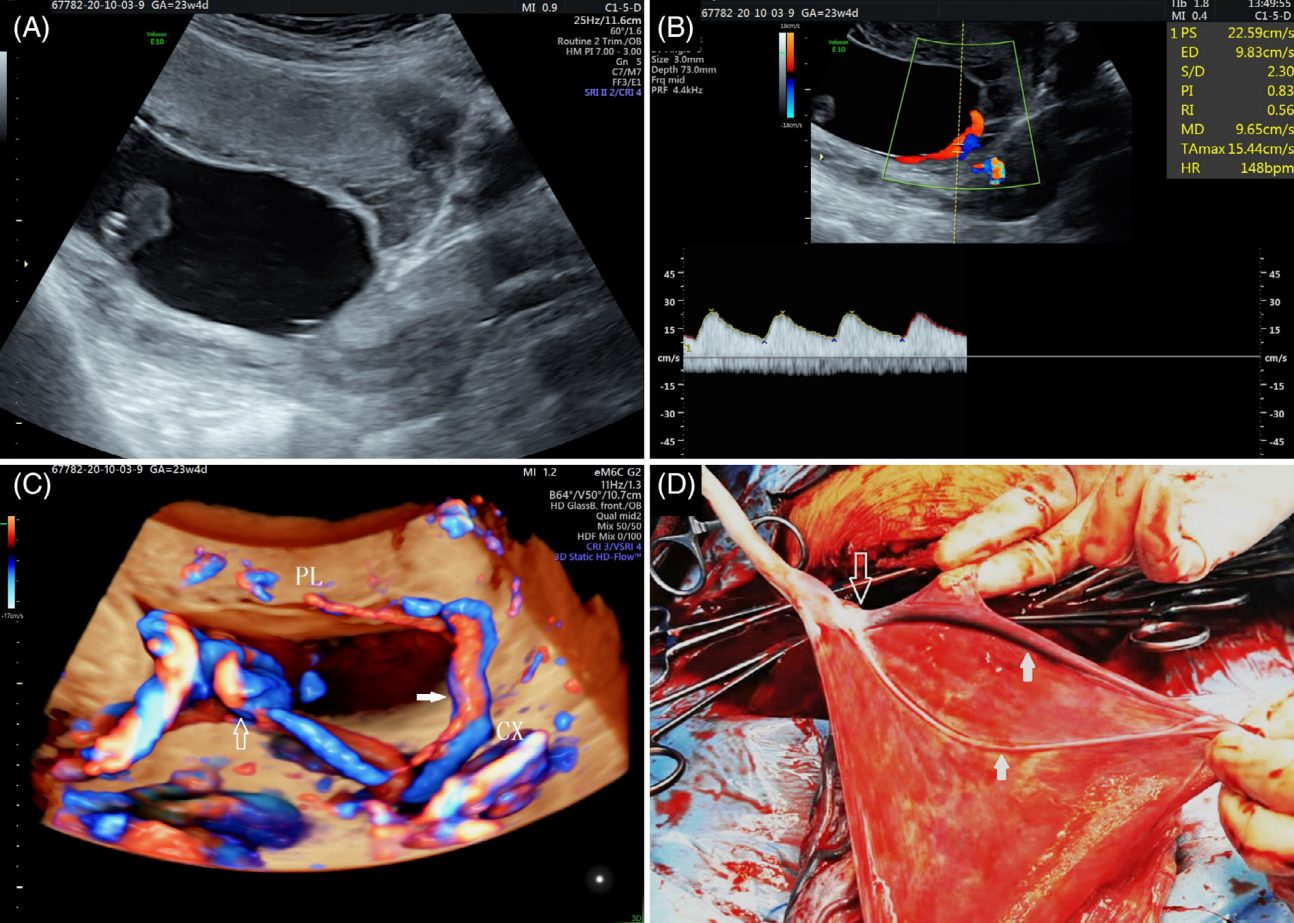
Two‐dimensional ultrasound examination showing short tubular hypoechoic structure near the cervical os suggestive of vessel previa (A). Color Doppler imaging demonstrating arterial and venous blood flow spectrum at the cervical os (B). Flow HD glass body of the umbilical cord showing sail‐like insertion and vessel previa crossing the cervical os and entering the placenta (C). PL, placenta; CX, cervix; hollow arrow, umbilical cord insertion point; solid arrow, sail‐like vessel. Postpartum image of placenta, umbilical cord, and umbilical vessels showing sail‐like insertion point (D). PL, placenta; hollow arrow, umbilical cord insertion point; solid arrow, sail‐like vessel.
